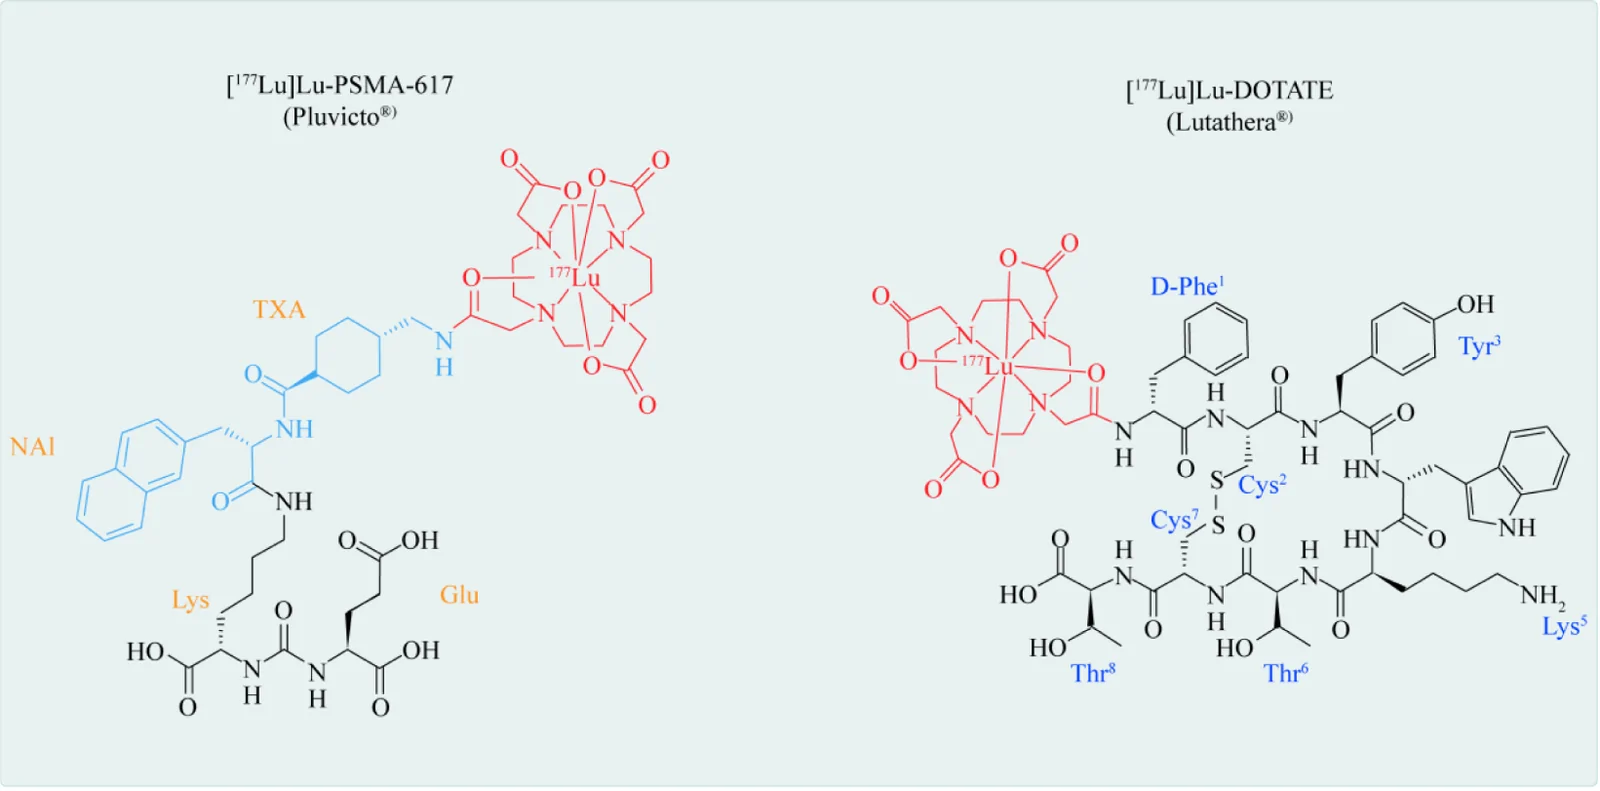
The molecular structures of Pluvicto® (^177^Lu-PSMA-617) and Lutathera® (^177^Lu-DOTA-TATE).
